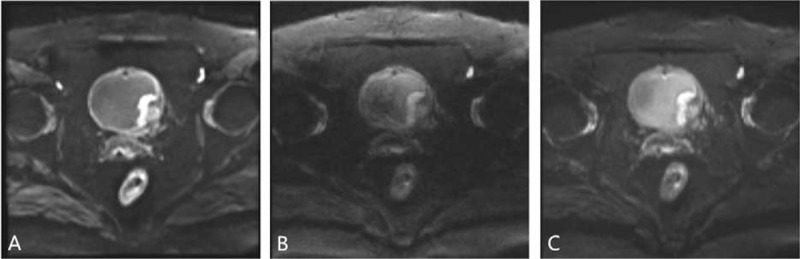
Comparison of (A) iShim, (B) RESOLVE, and (C) ss-EPI DWI (b = 1000 sec/mm^2^) in a 67-year-old female patient with bladder cancer. The lesion especially the edge of lesion and normal bladder wall can be seen more clearly on (A) iShim DWI. On (B) RESOLVE and (C) ss-EPI, the lesion edge and bladder wall obscured by image artifacts. Confidence score on (A) iShim, (B) RESOLVE, and (C) ss-EPI is 3, 3 and 3. DWI = diffusion-weighted imaging, iShim = integrated slice-specific dynamic shimming, RESOLVE = readout segmentation of long variable echo trains, ss-EPI = single-shot echo-planar-imaging.
